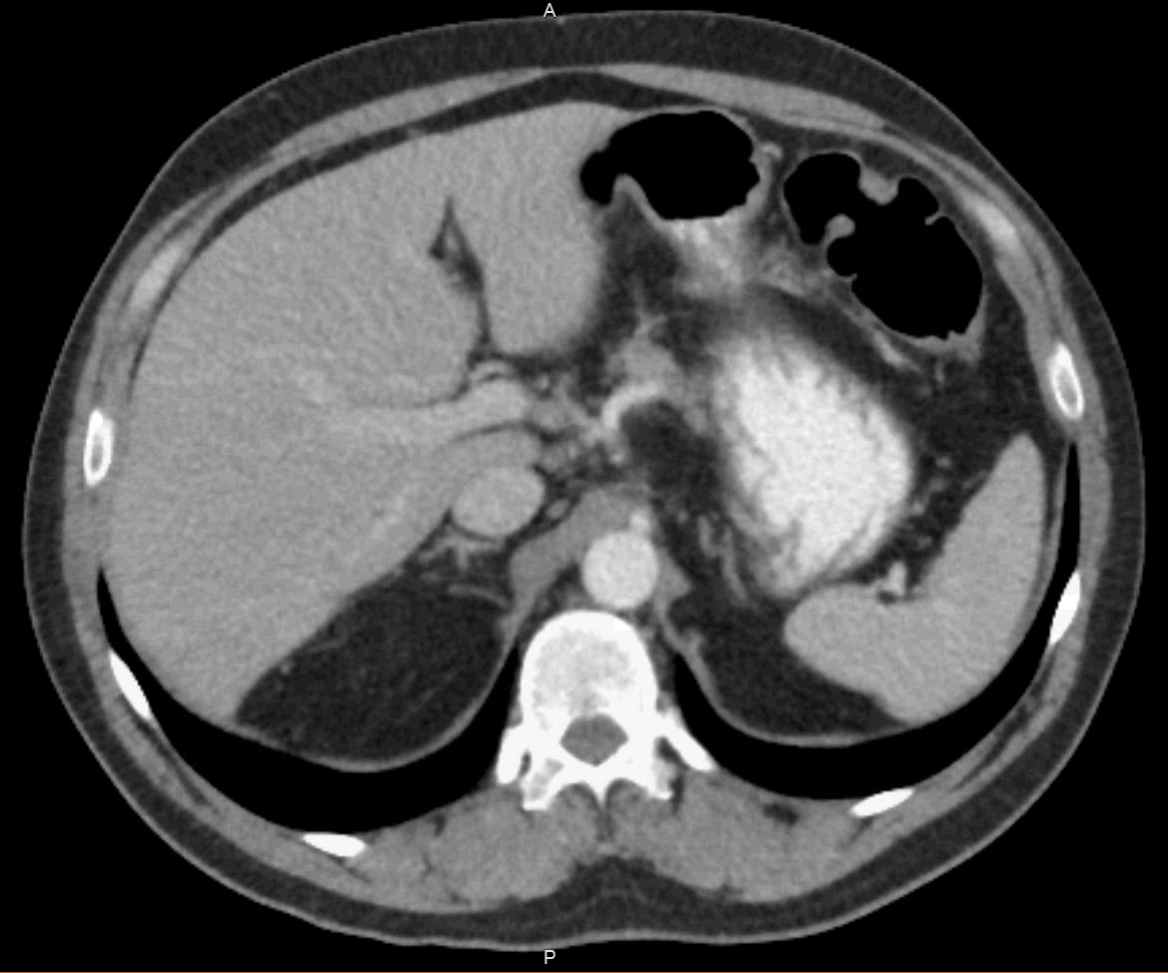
CT scan of abdomen after nine months CT: computed tomography
